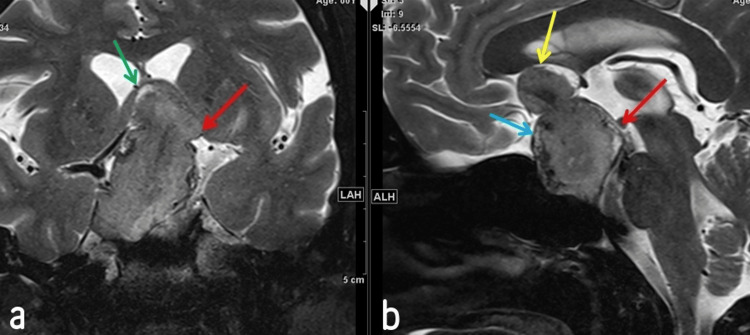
T2-weighted MRI in both coronal (a) and sagittal (b) views. These images reveal a sellar and large suprasellar mass with an intermediate signal intensity that elevates the third ventricle. The mass elevates the rostrum of the corpus callosum (yellow arrow). Furthermore, it abuts the mamillary bodies (red arrow). Peripheral calcification is also evident (blue arrow). Lastly, there is an elevation of the fornix (green arrow)
